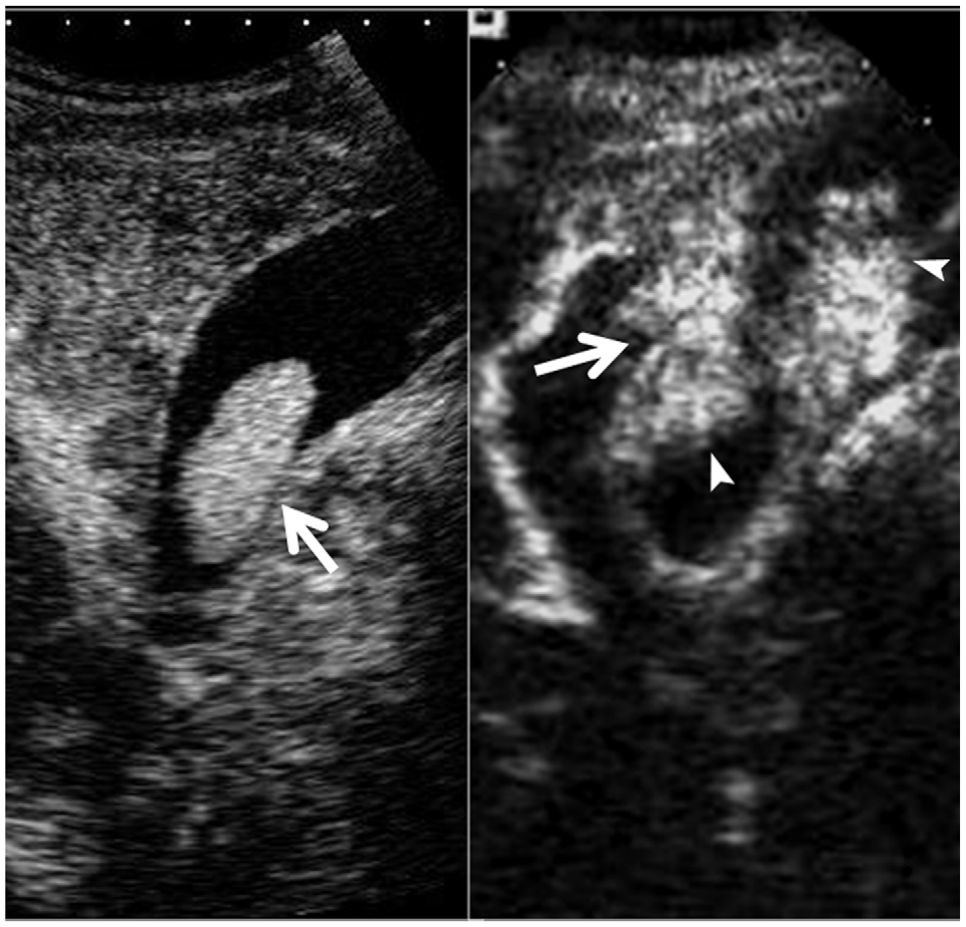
Intactness of the gallbladder wall on contrast-enhanced ultrasound. Left, the gallbladder wall (arrow) beneath an adenoma is intact. Right, the gallbladder wall (arrow) beneath a cancer (arrowheads) is destructed and the infiltration to the adjacent liver (arrow) is observed.
